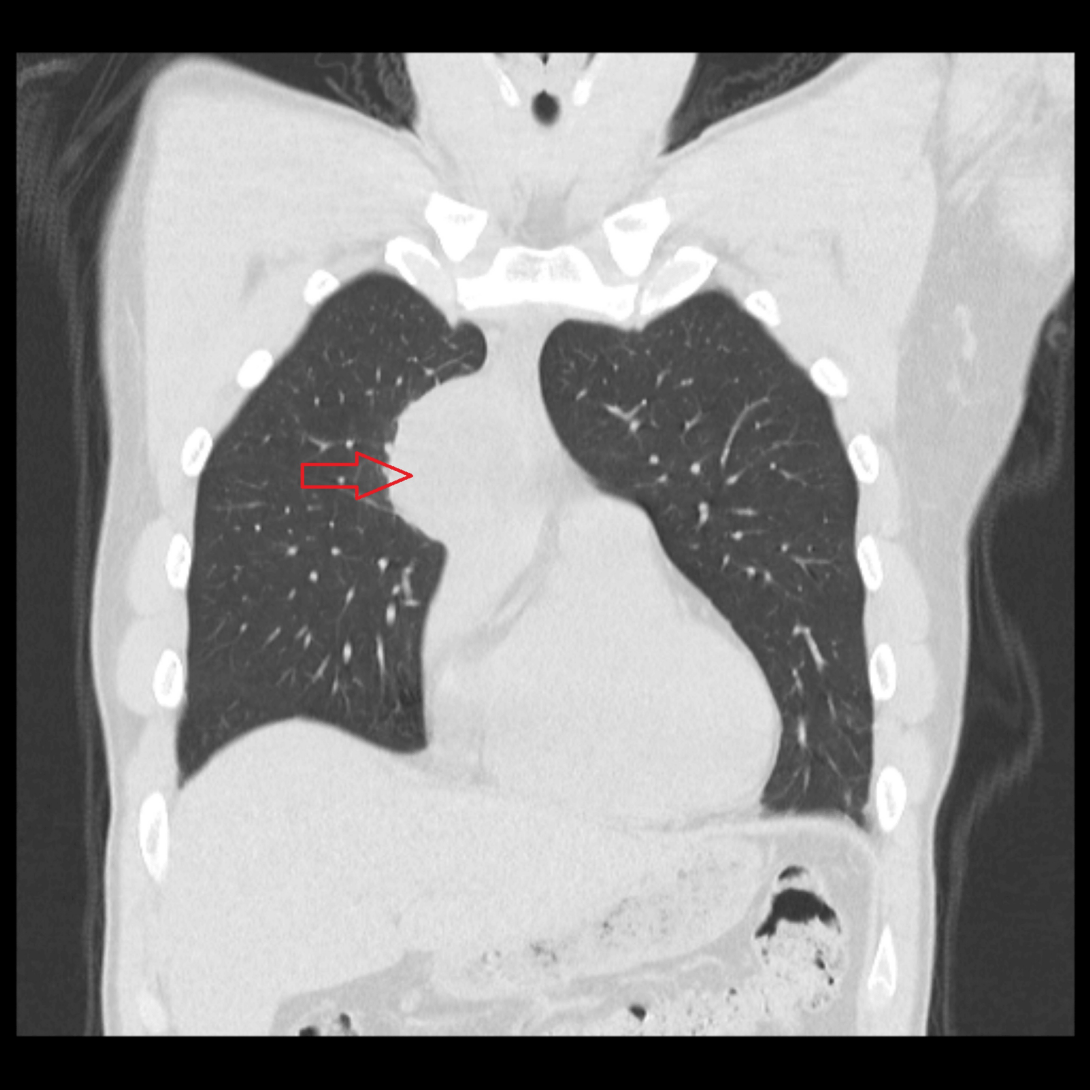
Coronal CT scan image exhibiting an anterior mediastinal mass and its relationship with surrounding structures
